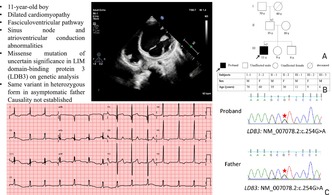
An 11‐year‐old boy presented with decompensated heart failure. Transthoracic echocardiogram showed severe biventricular dysfunction with an ejection fraction of 25% and left ventricular end‐systolic and end‐diastolic dimensions of 48 mm and 56 mm. No significant left ventricular hypertrophy was present.
